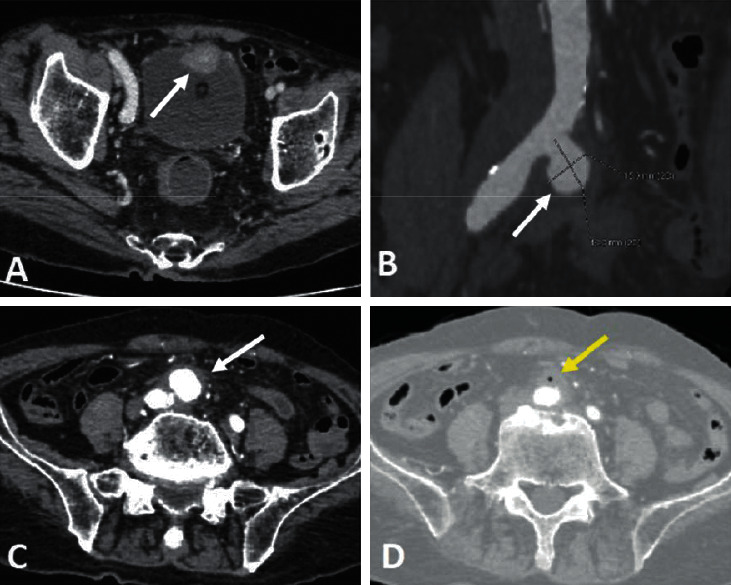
CT scanner forty days prior to admission. (a) Enhanced axial CT shows a bladder tumor (arrow). (b, c) Enhanced coronal and axial CT shows a 17 × 18 mm saccular aneurysm arising from the right common iliac artery (arrows) surrounded by fat stranding. (d) Enhanced axial CT shows small foci of gas (arrow).
